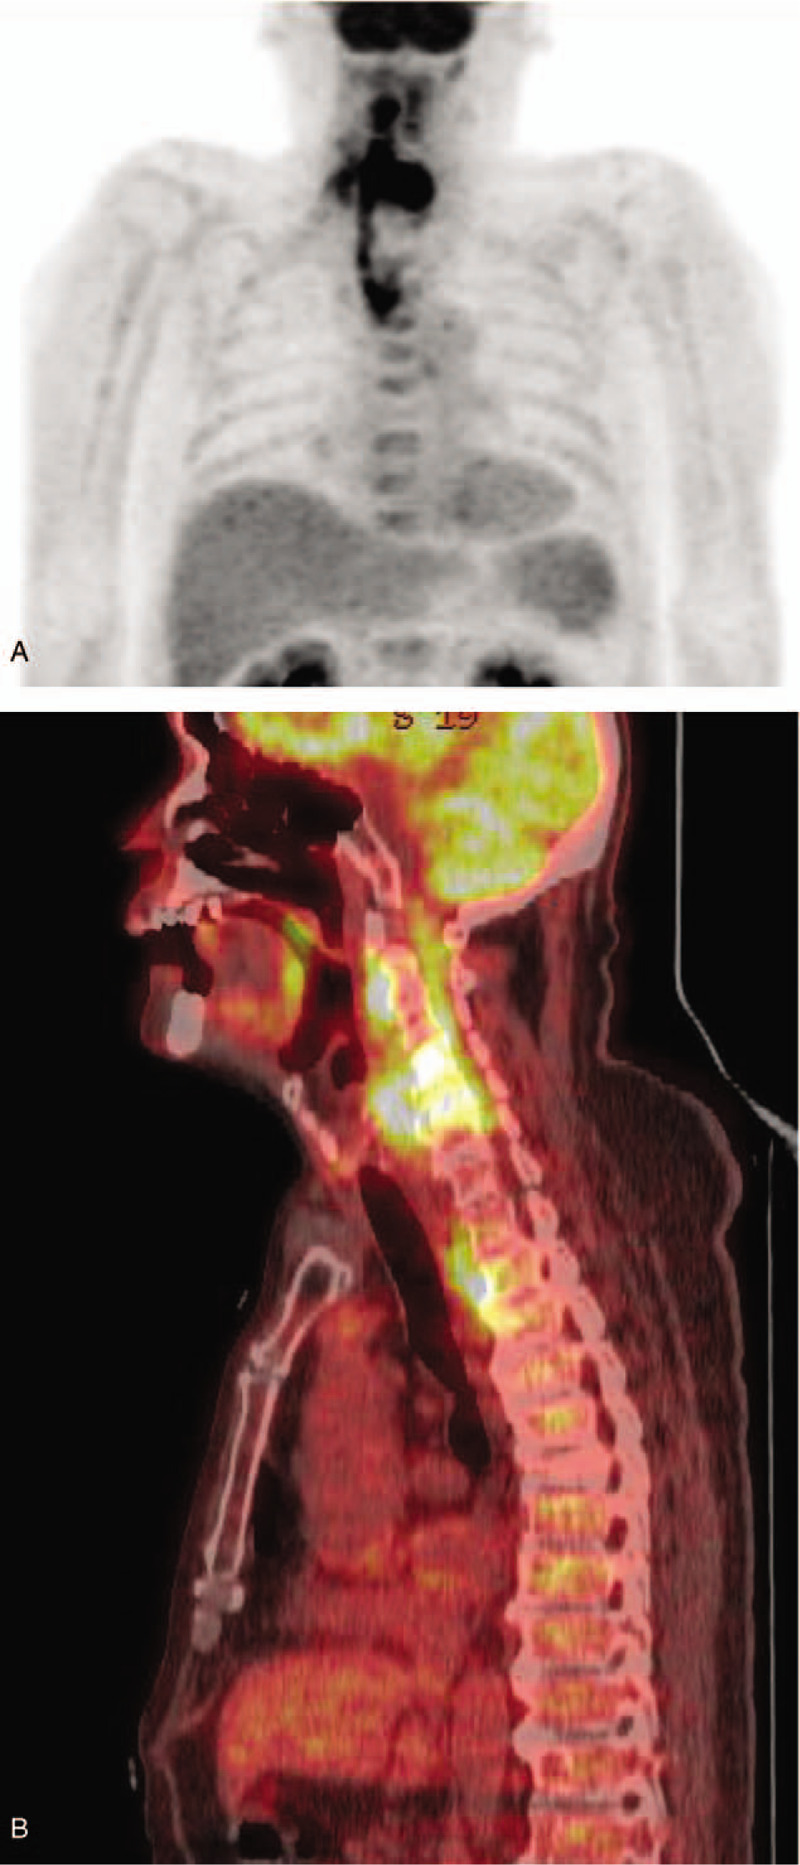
The maximum intensity projection image of positron emission tomography (A) and sagittal scan of positron emission tomography/computed tomography fusion imaging (B) reveal continuous hypermetabolic lesions along the prevertebral and danger spaces of the neck from the C2–T4 levels with associated osteomyelitis of the vertebral bodies of C4 and C5.
